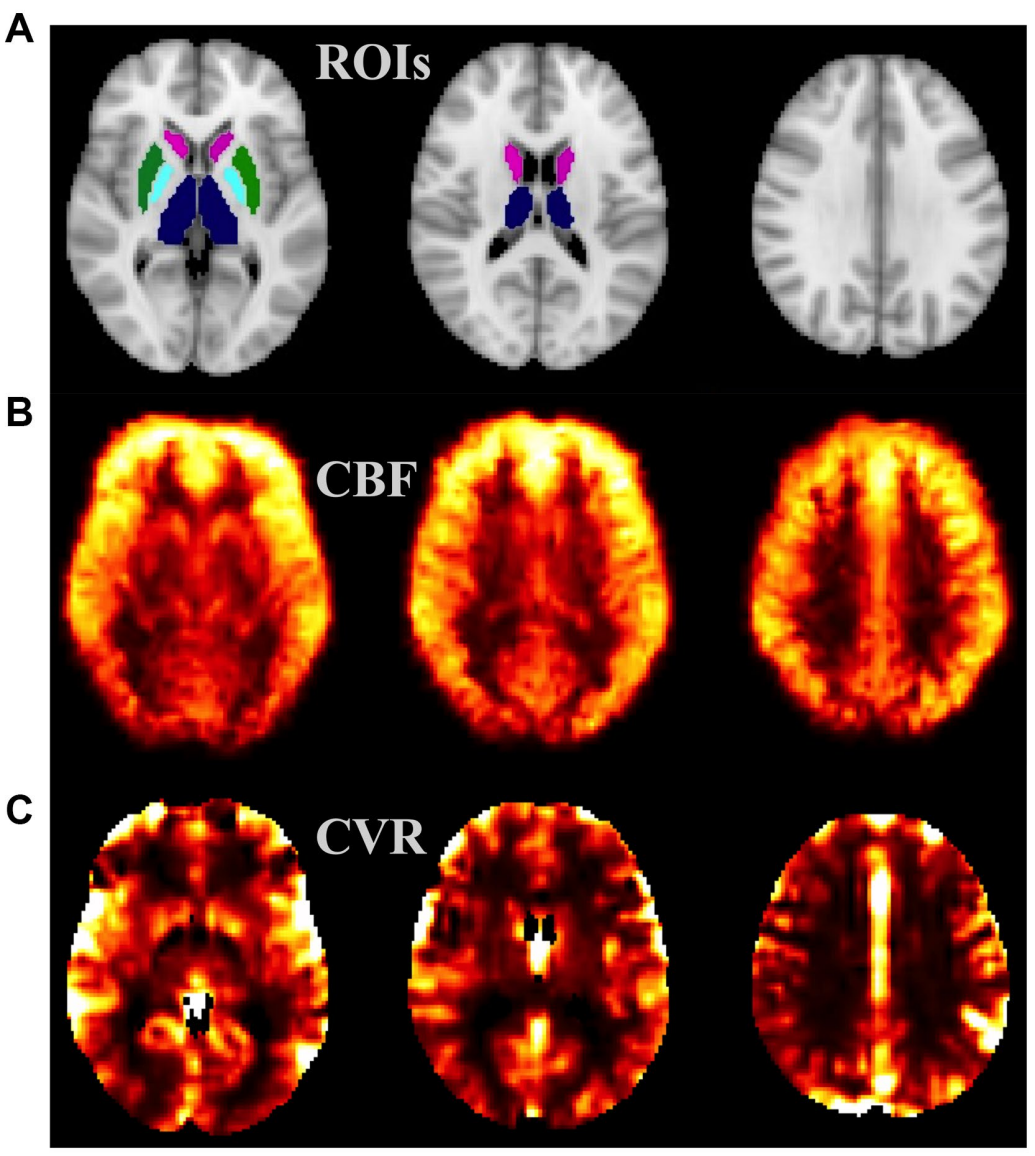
Example MRI maps from a healthy subject: (A) ROIs considered—TH (blue), CN (magenta), PUT (green), and GP (cyan); (B) cerebral blood flow (CBF) map, intensity scale: 0–50 mL/100 g/min; (C) cerebrovascular reactivity (CVR) map, intensity scale: 0–3 relative unit. CN, caudate nucleus; PUT, putamen; GP, Globus Pallidus; ROI, region of interest.
